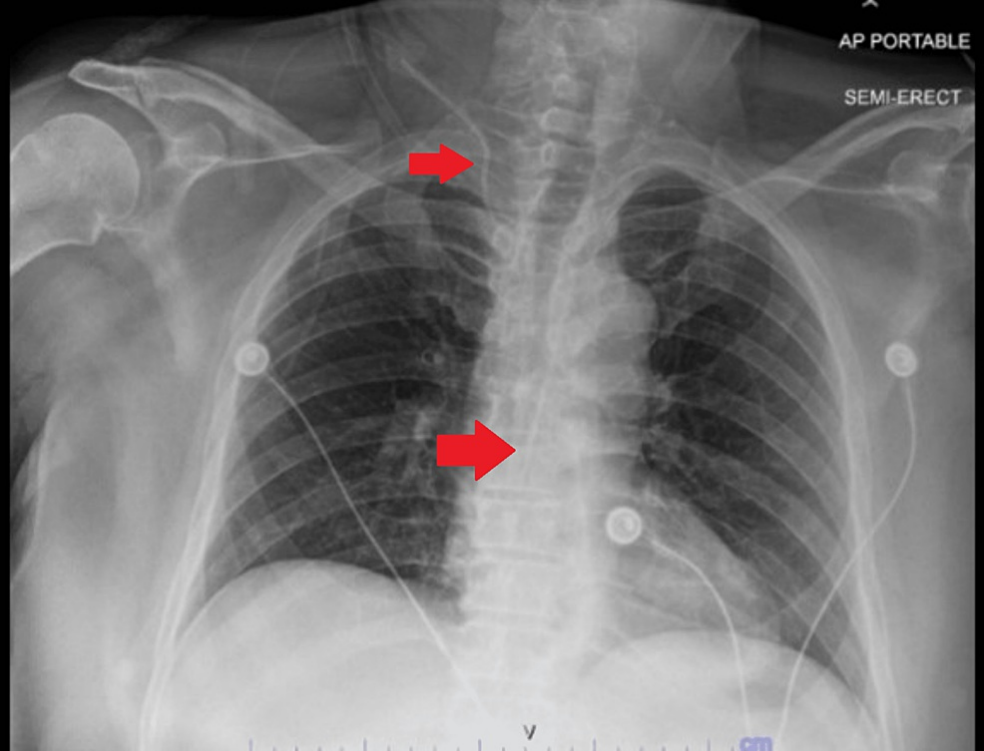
Chest AP radiograph confirming central venous catheter placement in the right internal jugular vein
